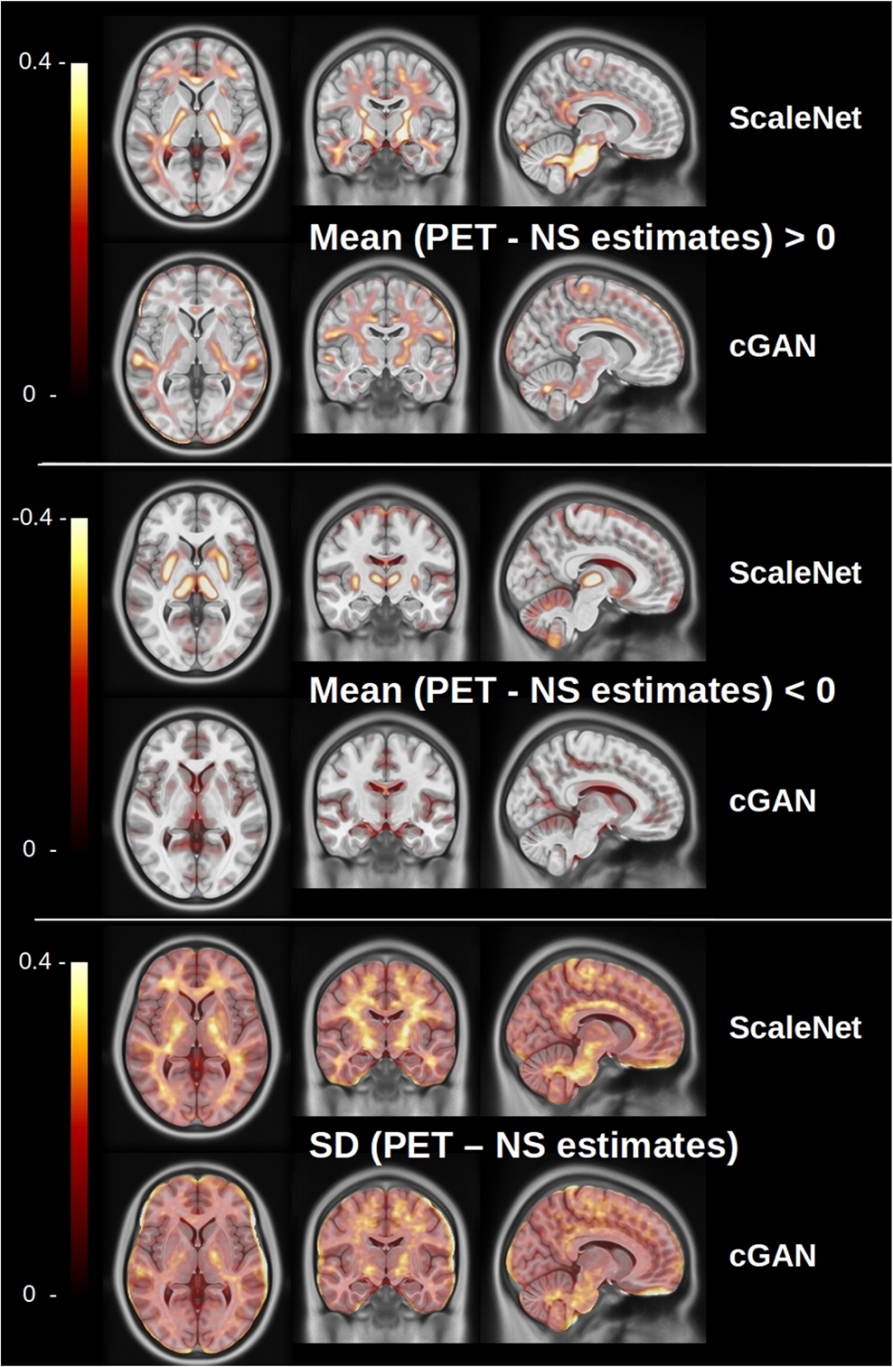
Mean SUVr absolute difference and standard deviation images computed in MNI-space from the 40 training PET scans and the corresponding NS images estimated with ScaleNet and cGAN. Top: regions where the NS is underestimated, middle: regions where the NS is overestimated, bottom: standard deviation images
